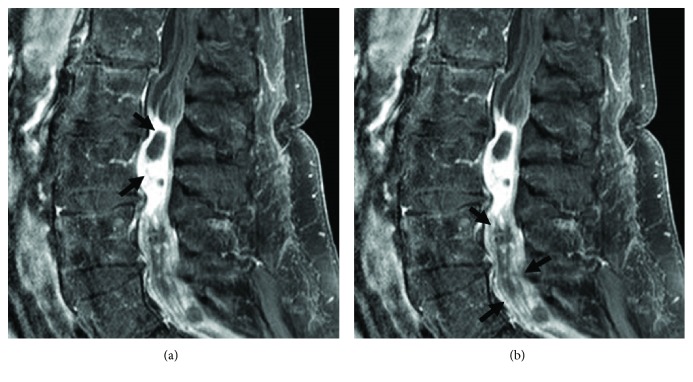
Magnetic resonance imaging (MRI) study was performed one month after onset of symptoms and showed (a) a lesion with extensive enhancement of the lesion periphery and (b) extension of enhancement to the distal nerve roots on sagittal fat-suppressed T1-weighted MRI.
